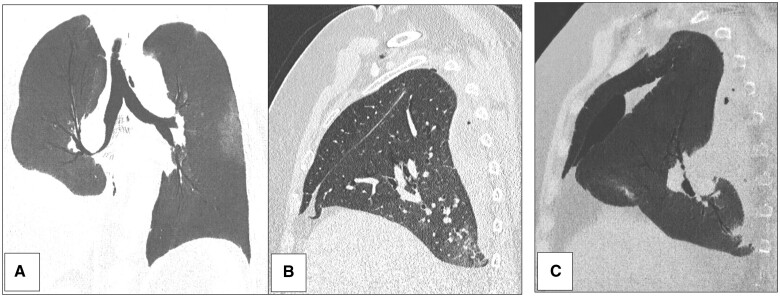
Case no 1: chest CT scan at day 7 with coronal view (A) and sagittal views (B and C). A middle and lower lobe malposition with a 180° lobar tilt were revealed. Middle lobe was in back position. Diaphragmatic face of right lower lobe was close to back part of anterior chest wall and Fowler’s segment was in extreme lower part of the pleural cavity.
